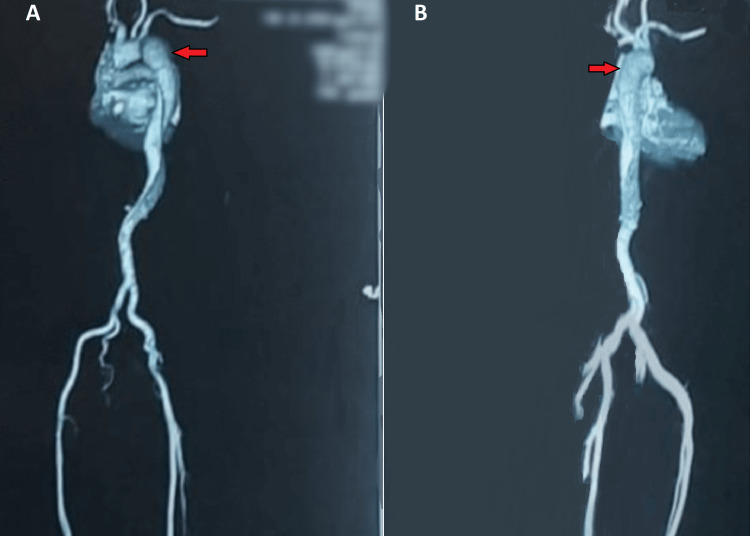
(A) CT abdominal angiography anteroposterior view showing aortic dissection distal to the left subclavian artery; (B) CT abdominal angiography lateral view showing aortic dissection
